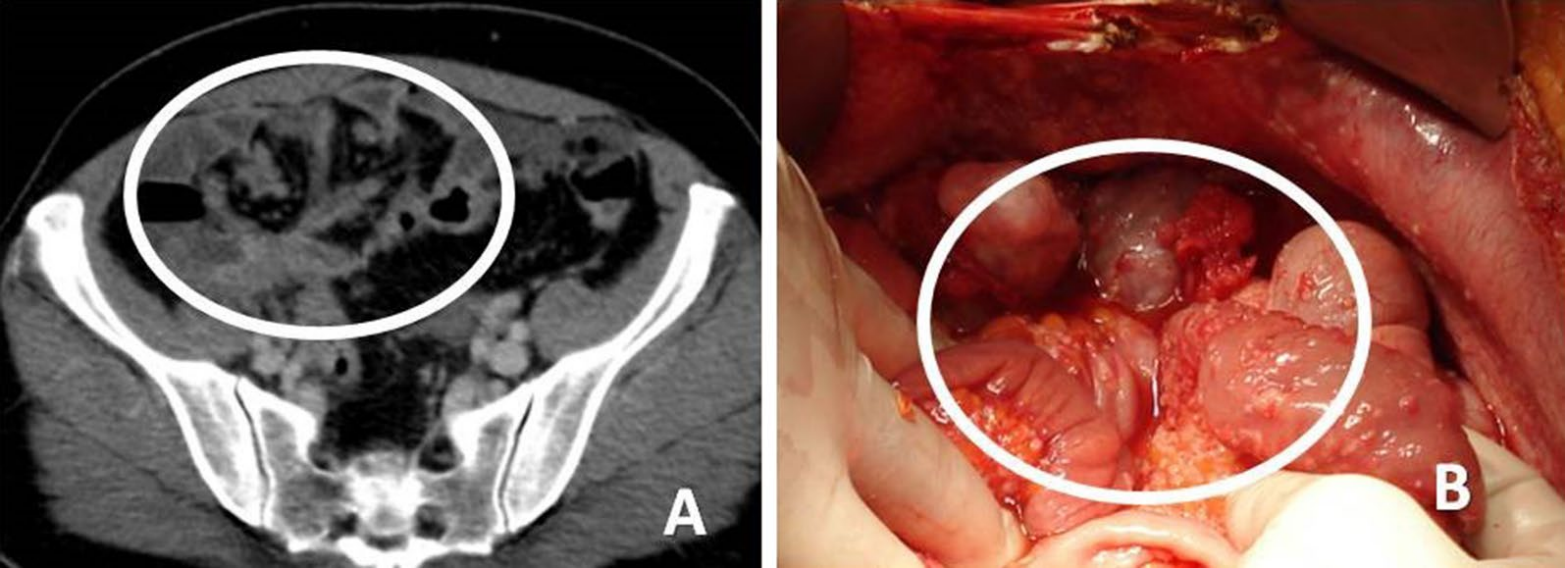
a Contrast enhanced CT enteroclysis image shows non dilated intestinal loops due to rigidity caused by multiple tiny implants on the surface of the intestinal loops (white circle). b At surgery shorted and distorted intestinal loops with numerous deposits are seen (white circle)
